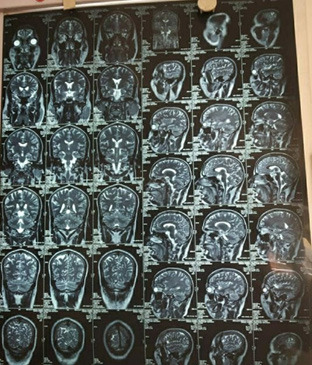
MRI of brain showing T2 and flair images showing left subacute thrombus noted in transverse sinus.
